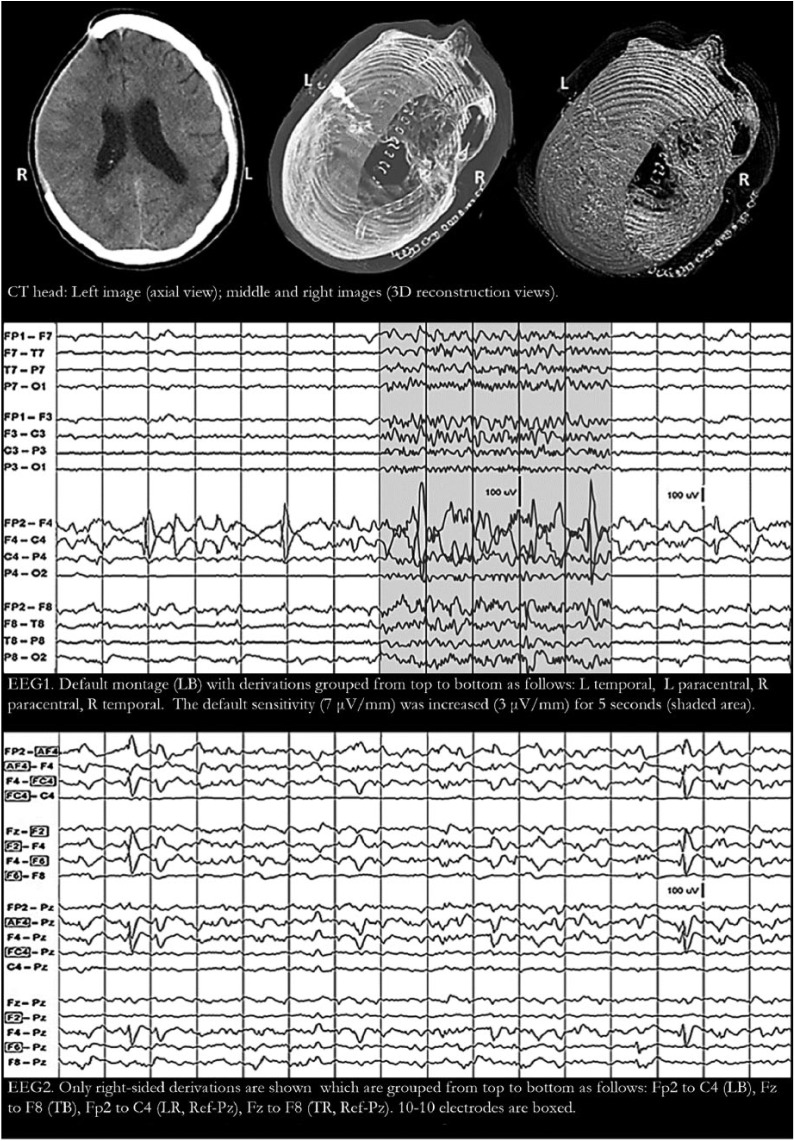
Patient 1 has a large right frontotemporal craniectomy with absent bone flap (CT [computed tomography] head). Electroencephalogram (EEG) monitoring showed phase reversals in FP2-F4 and F4-C4 consistent with F4 electrode artifacts (EEG1). Increasing display sensitivity showed an occasional hint of a physiological field but this was not enough to rule out electrode artifact (EEG1 shaded area). Transferring 4 electrodes from the left side of the head to 10-10 electrode locations around F4 (AF4, FC4, F2, and F6) made it clear that the F4 potentials have some type of physiological field (EEG2). For all EEG tracings above: filters at 1-Hz high-pass, 70-Hz low-pass, and 60-Hz notch; 1 second/division; and100-µV voltage scale shown in tracing. L, left; R, right; Ref, reference; LB, longitudinal bipolar; TB, transverse bipolar; LR, longitudinal referential; TR, transverse referential.
